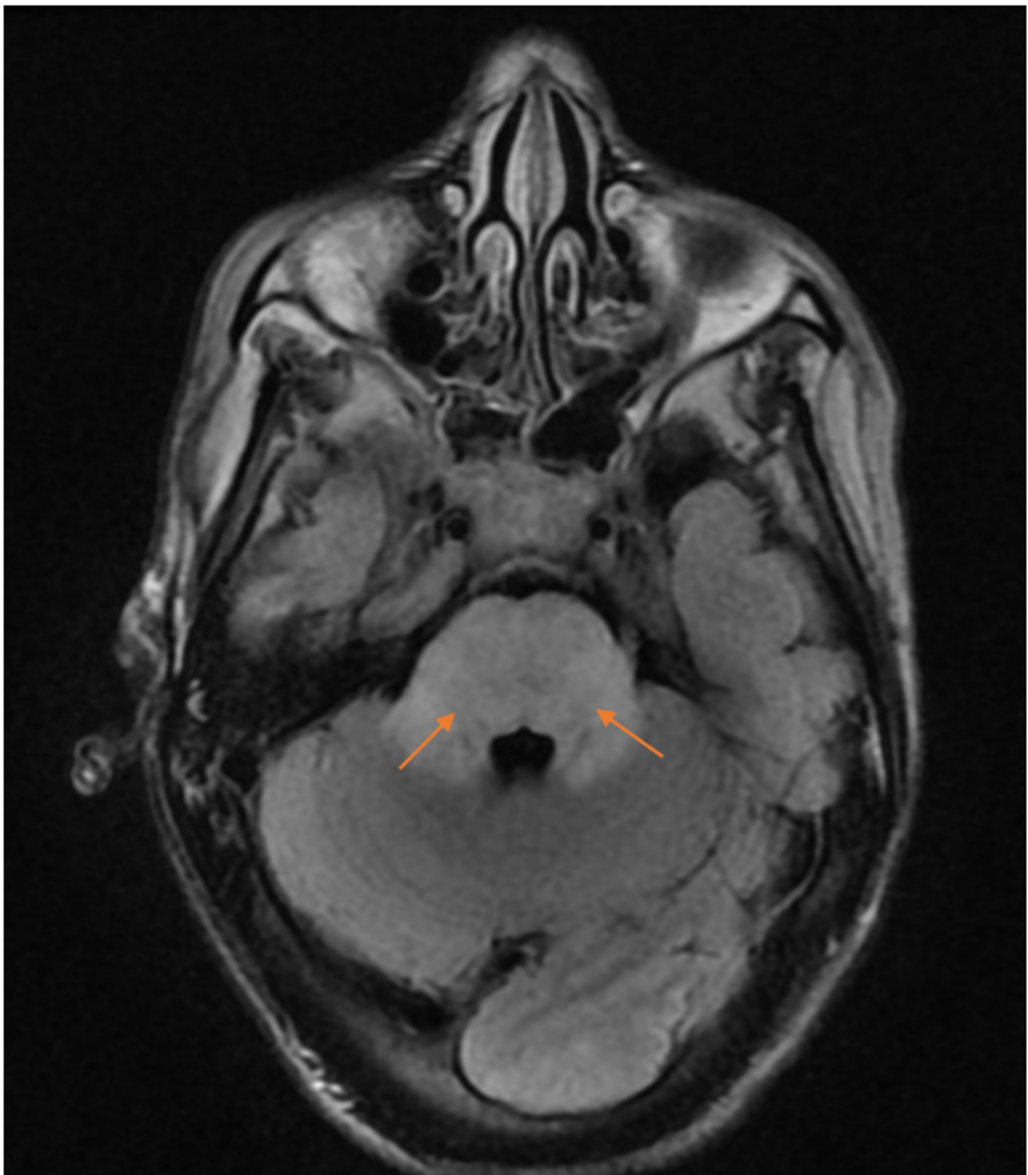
T2 MRI image of the brain showing multifocal T2 hyperintensity within the white matter bilaterally in a fairly symmetric distribution (arrows). Extensive T2-hyperintensity within the midbrain and pons involving the cerebellar peduncles is seen due to demyelinating processes.
